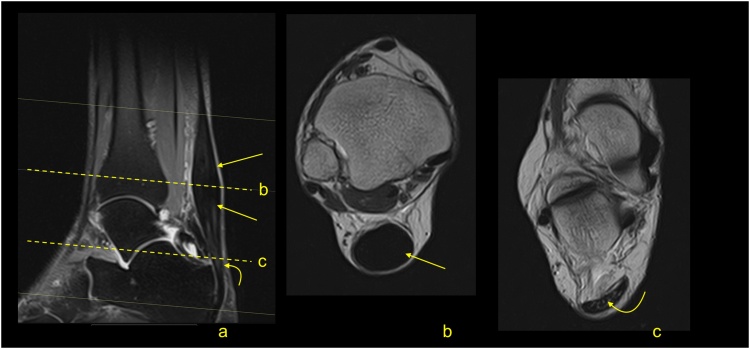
Midportion tendinopathy of the Achilles tendon. The MRI (a–c) revealed the thickening of the Achilles tendon (straight arrow) in the midportion, while the insertion is regular (curved arrow). A- T2-weighted with fat suppression, b and c- PD-weighted.
